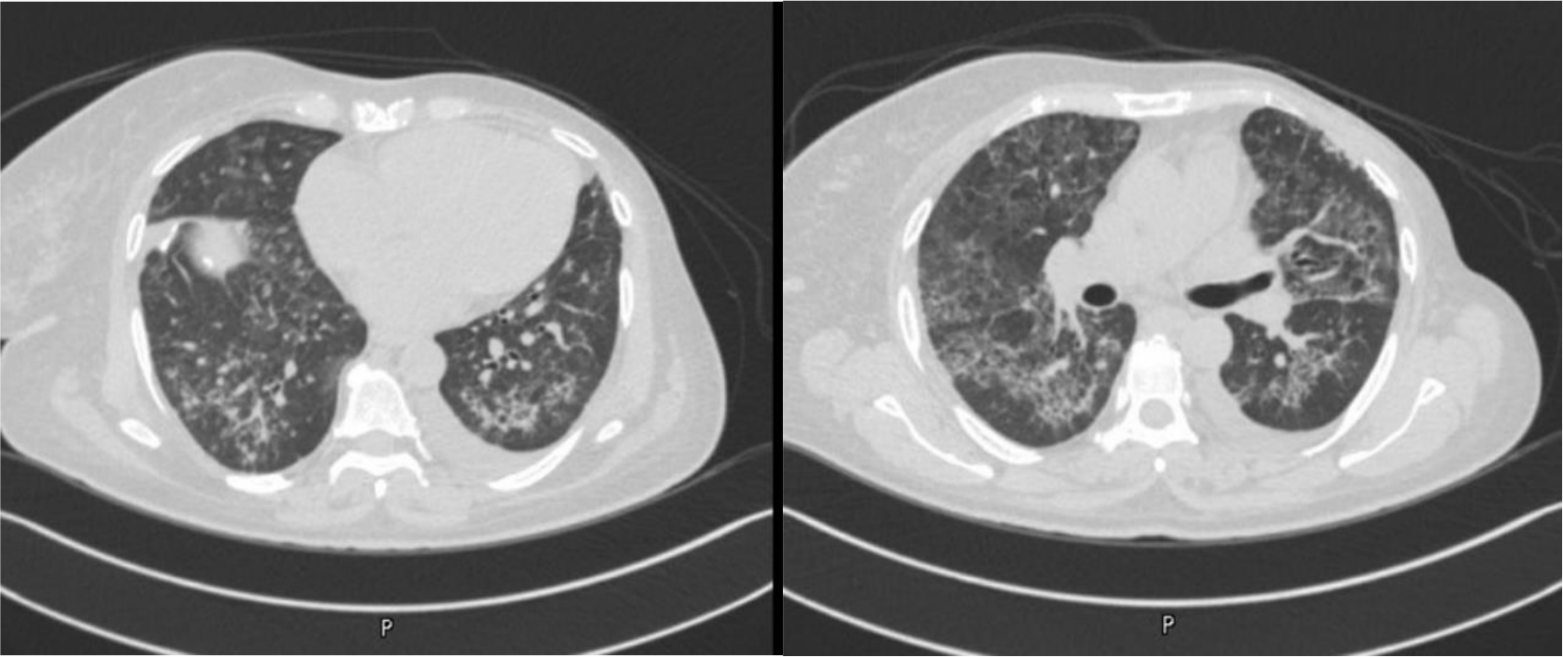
Chest CT scan showing progression of GGOs compared to Figure 1.
